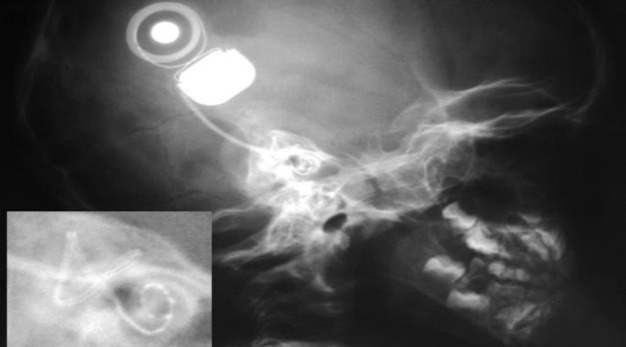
Postoperative X-ray confirming the position of the electrode array into the basal turn of the cochlea (the inset image is a magnification showing the electrode in the basal turn of the cochlea).
